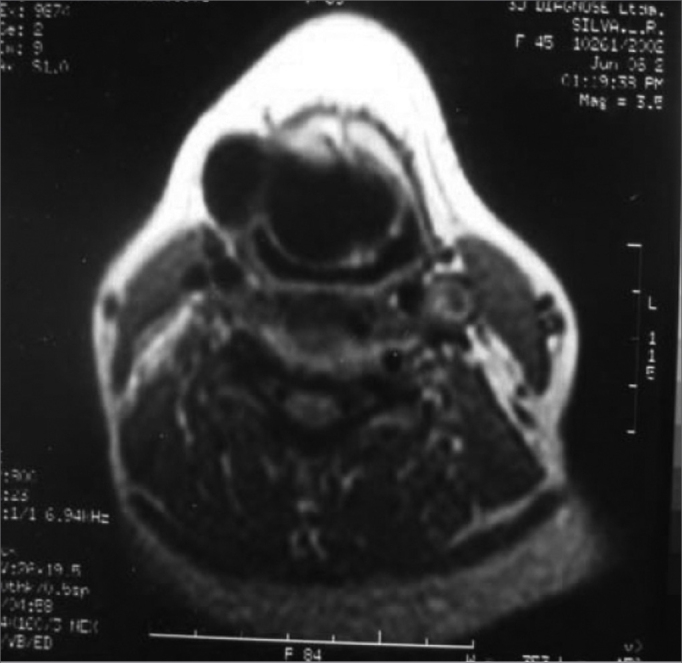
MRI showing the laryngocele in details.
